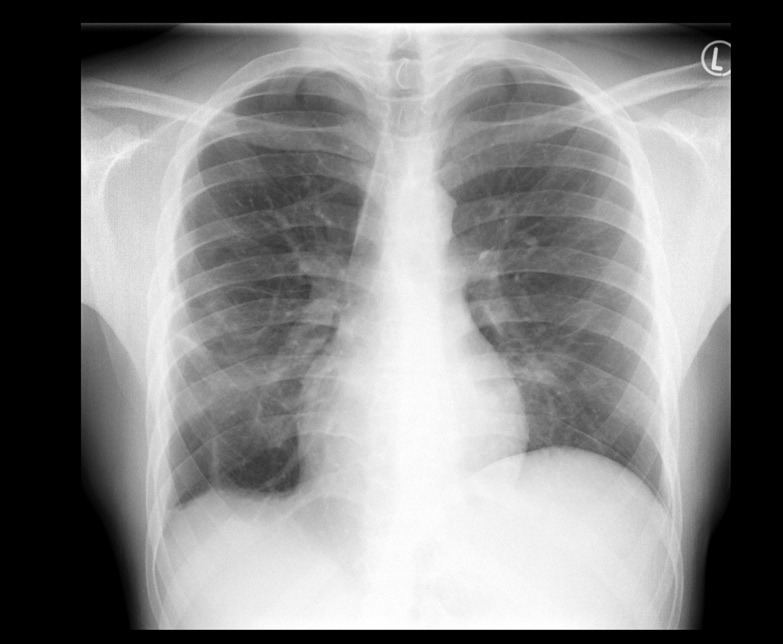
Chest radiograph 2 months post bilateral thoracotomy
